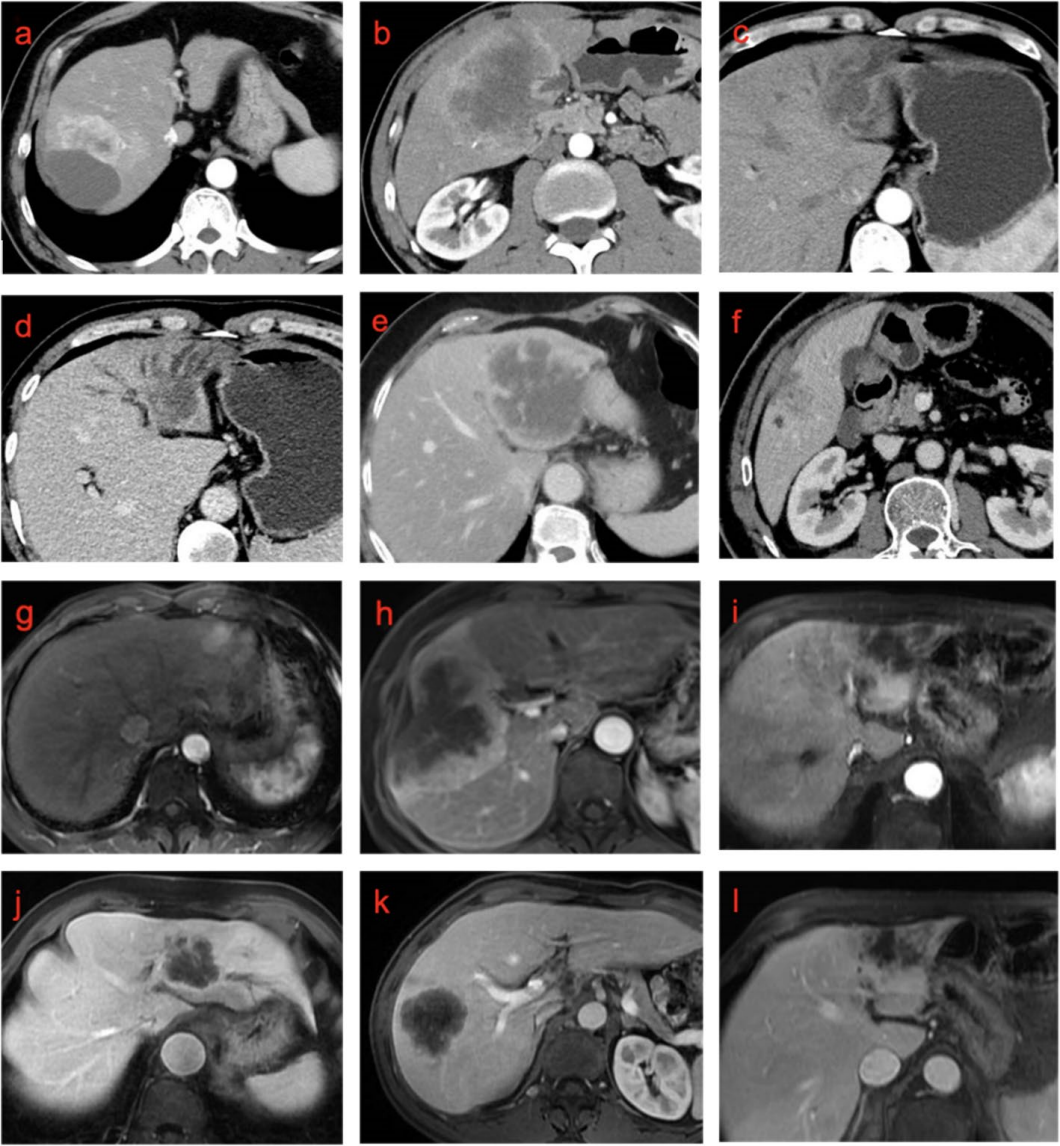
Examples of imaging features of patients with ICC. a Hyperenhanced ICC in CT images. b Rim-enhanced ICC in CT images. c Hypoenhanced ICC in CT images. d Bile duct invasion in CT images. e Clear tumor margin in CT images. f Infiltrative tumor margin in CT images. g Hyperenhanced ICC in MRI images. h Rim-enhanced ICC in MRI images. i Hypoenhanced ICC in MRI images. j Bile duct invasion in MRI images. k Clear tumor margin in MRI images. l Infiltrative tumor margin in MRI images
